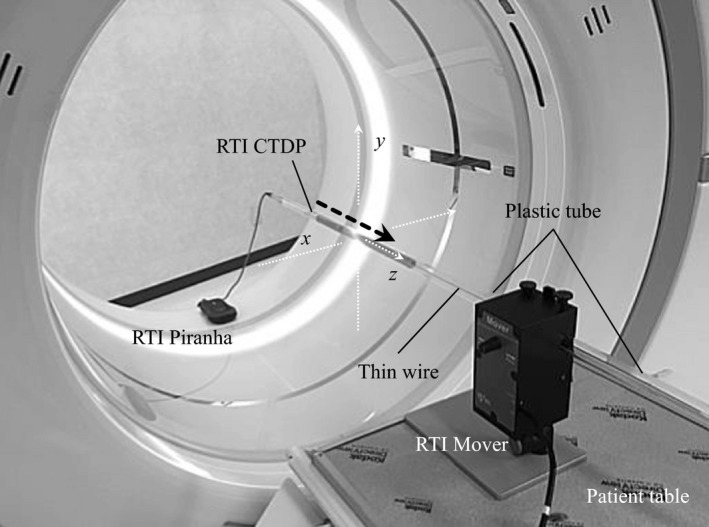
Photograph of the experimental setup for dose profile measurements. The small sensor embedded in the computed tomography dose profiler probe was moved at a constant speed of 83 mm/s by the RTI Mover during continuous use of the 160‐mm non‐helical volume‐acquisition mode.
